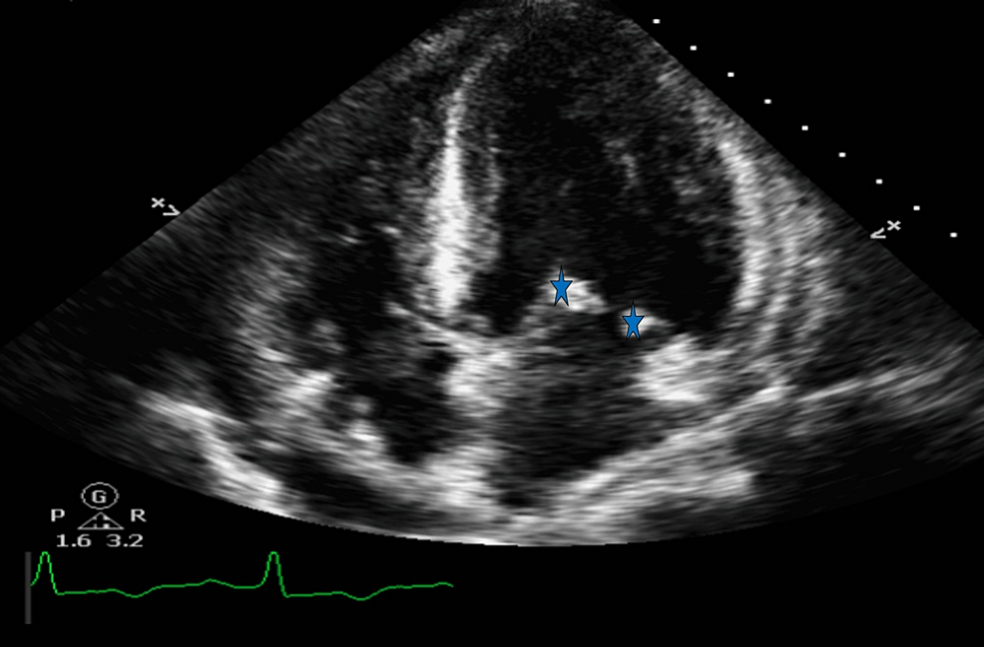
Transthoracic echocardiogram in the four-chamber view depicted Libman-Sacks endocarditis on the mitral valve (blue stars)
